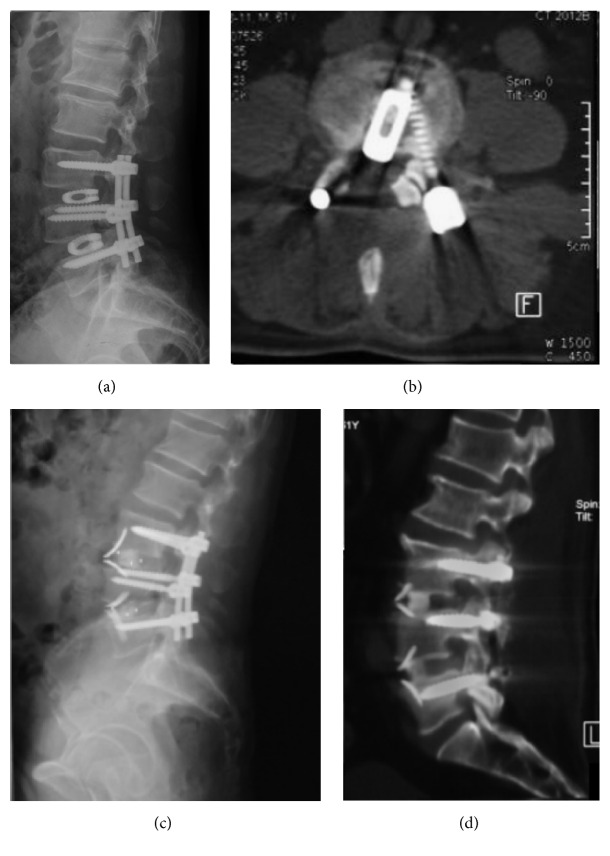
Case presentation. A 61-year-old male patient with previous PLIF surgery at L3-L5 10 months ago was admitted because of the recurrence of back and leg pain. Cage migration was confirmed by both radiograph and CT scan ((a) and (b)). ALLIF revision surgery using a self-anchored cage was performed. Good position of cage levels and satisfactory alignment of the lumbar spine were achieved (c). Fusion was achieved at 12-month follow-up (d).
